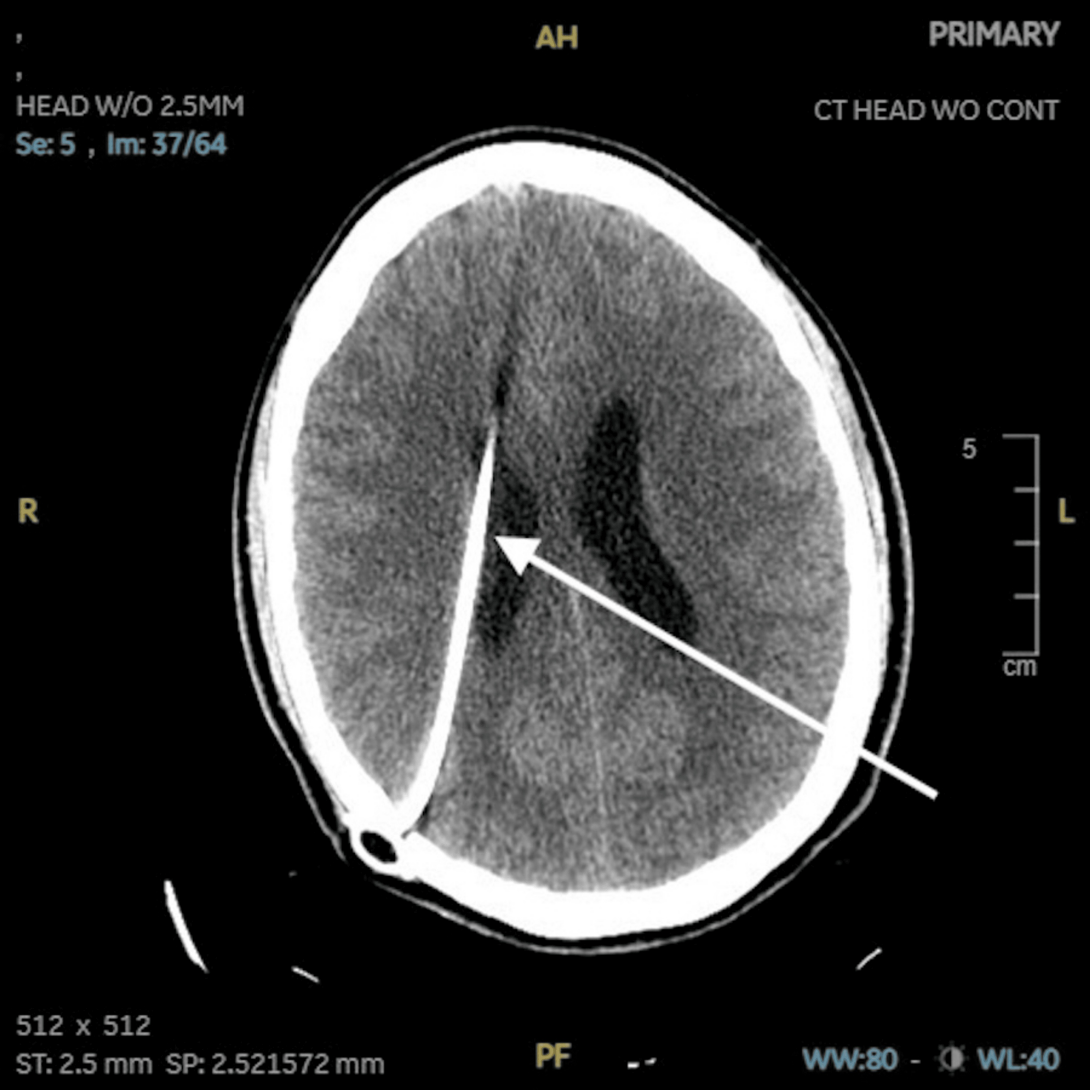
CT scan of the head axial view demonstrating a VP shunt and improved ventricular dilation and hydrocephalus CT: computed tomography; VP: ventriculoperitoneal
